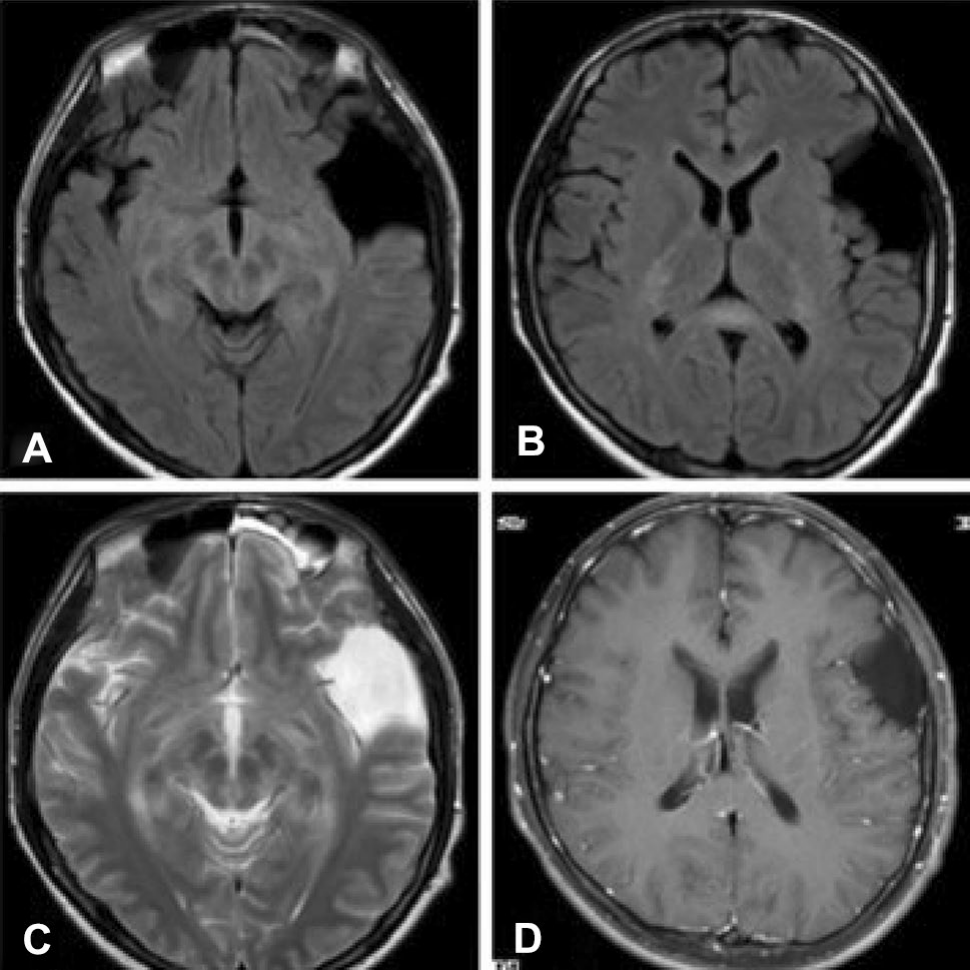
1 MRI of case 1: FLAIR visible hippocampal, thalamic, and midbrain high signal (A); callosal compression lesion (B); T2 sequence visible hippocampal, thalamic, and midbrain high signal (C); T1 enhancement visible as a discharge-like strengthening sign a discharge-like strengthening sign around the lateral ventricles, with no signal strengthening in the corpus callosum (D) (note: the patient had a similar lamellar high signal in the cervical medulla T2)
